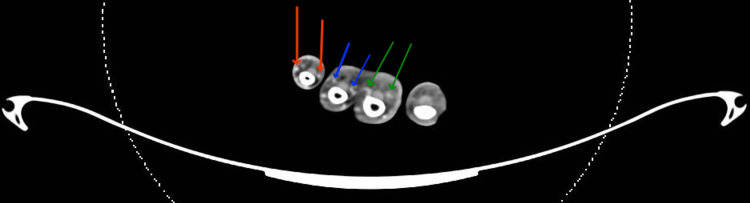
CT angiography The patency of the proper digital arteries in the little, ring, and middle fingers, while demonstrating obliteration of the proper digital arteries of the index finger. Indicators of the proper digital arteries for the little, ring, and middle fingers are red, blue, and green arrows, respectively.
